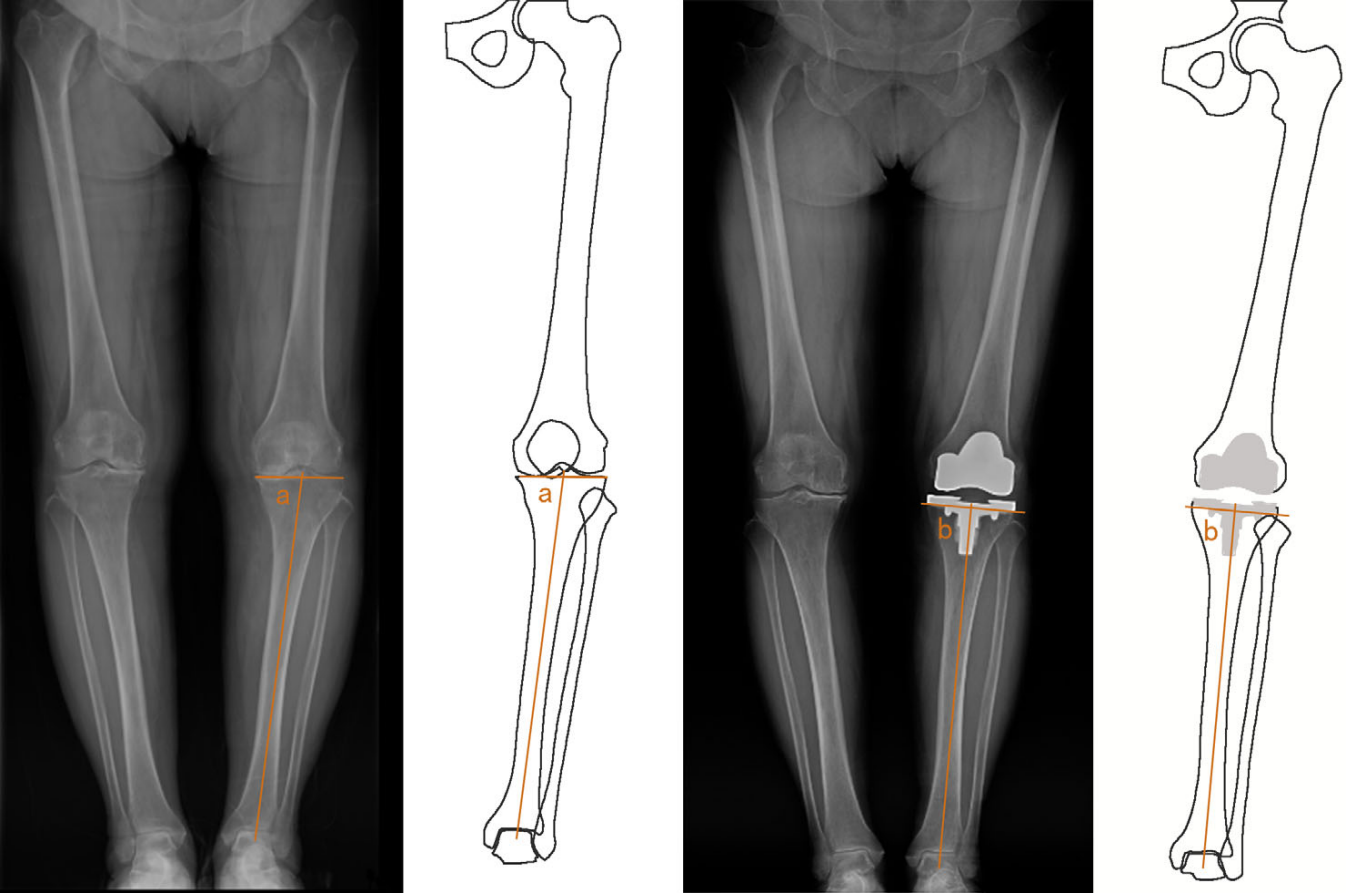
Radiograph showing the measurement of MPTA. MPTA is the medial angle formed by the tibial mechanical axis and frontal plane joint line of the proximal tibia (MPTA before and after surgery).
